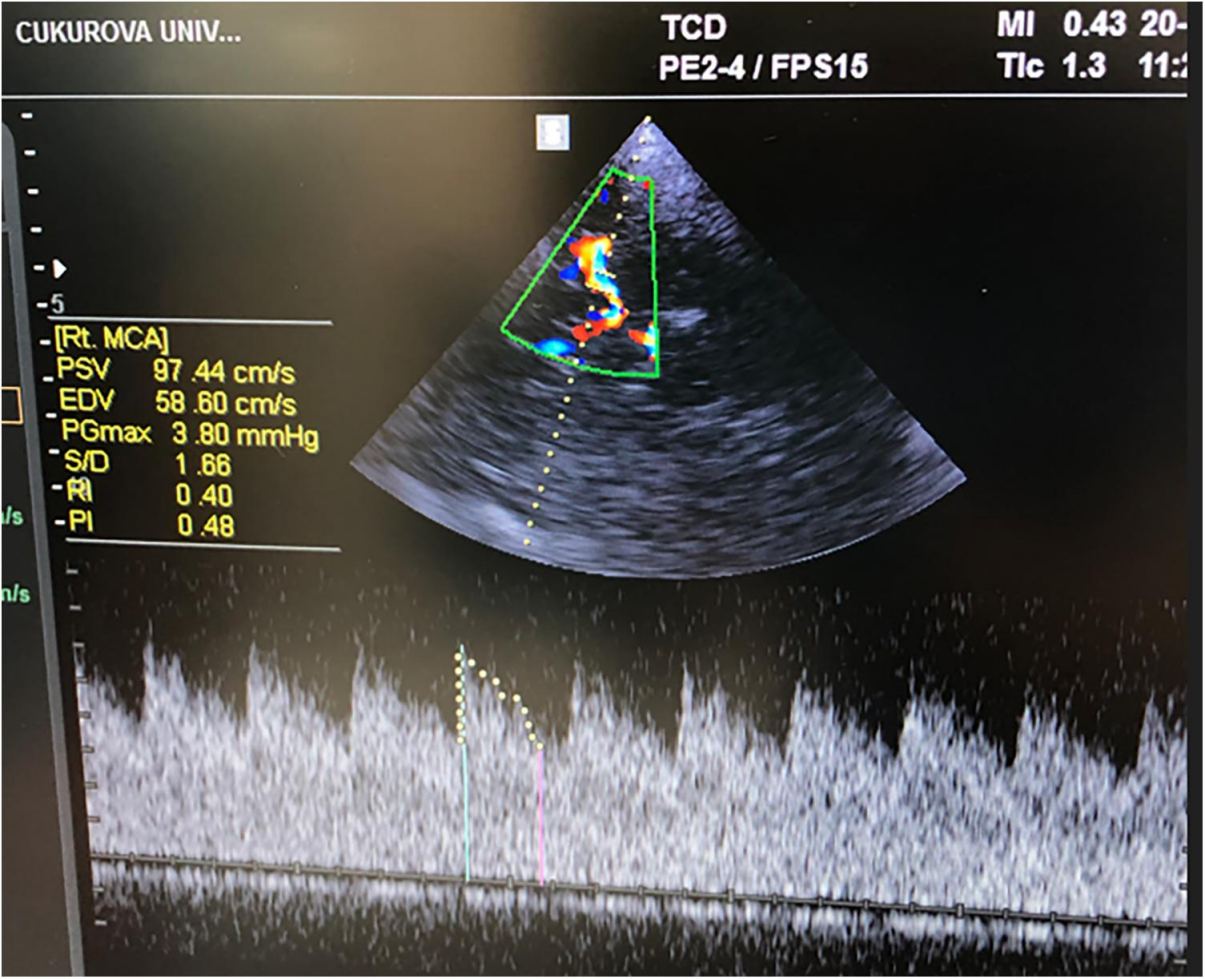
TCD MCA blood velocities and Doppler indices.
